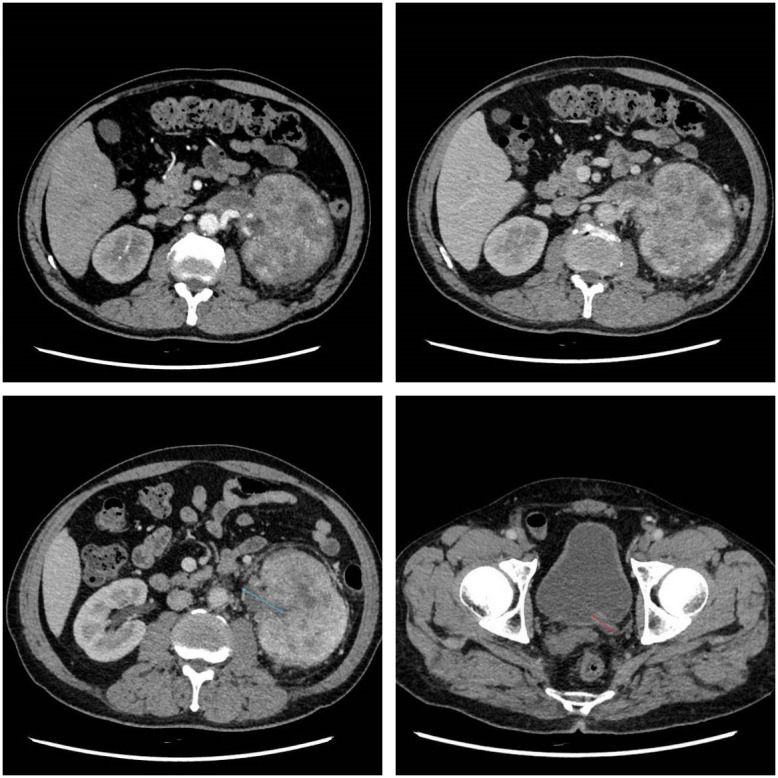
The blue arrow indicates the tumor thrombus within the renal pelvis and proximal ureter. The red arrow shows the ureteral tumor thrombus under CT.
